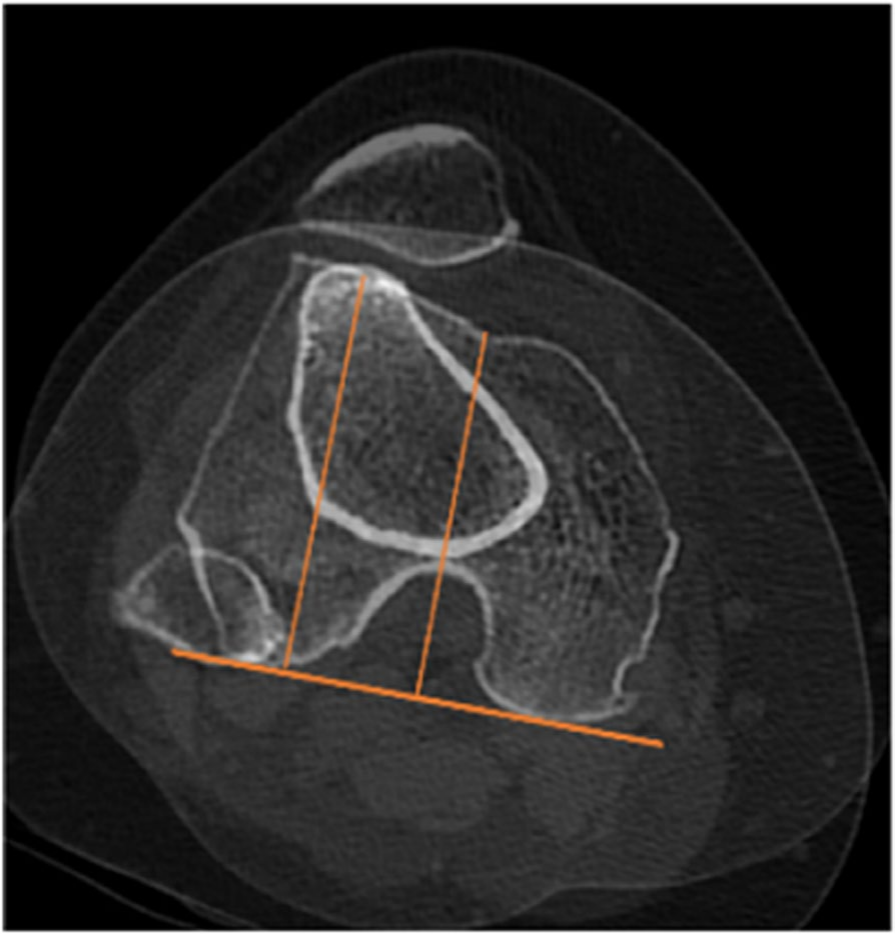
The TT-TG distance is defined as the distance measured on CT between the most anterior part of the tibial trochanter and the middle of the trochlear groove
